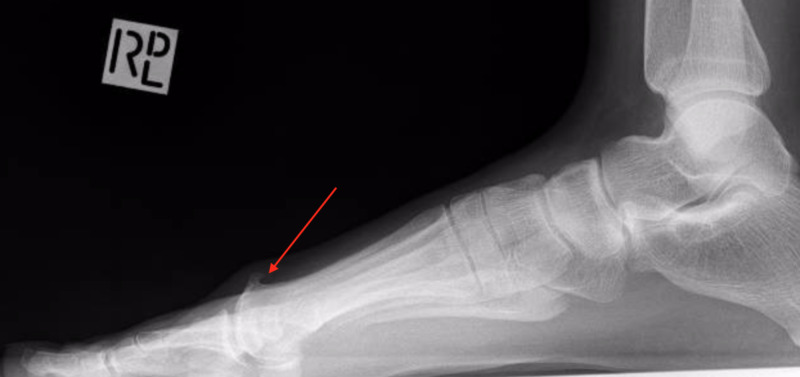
Lateral x-ray showing the dorsal osteophyte formation overlying the head of the first metatarsal.
